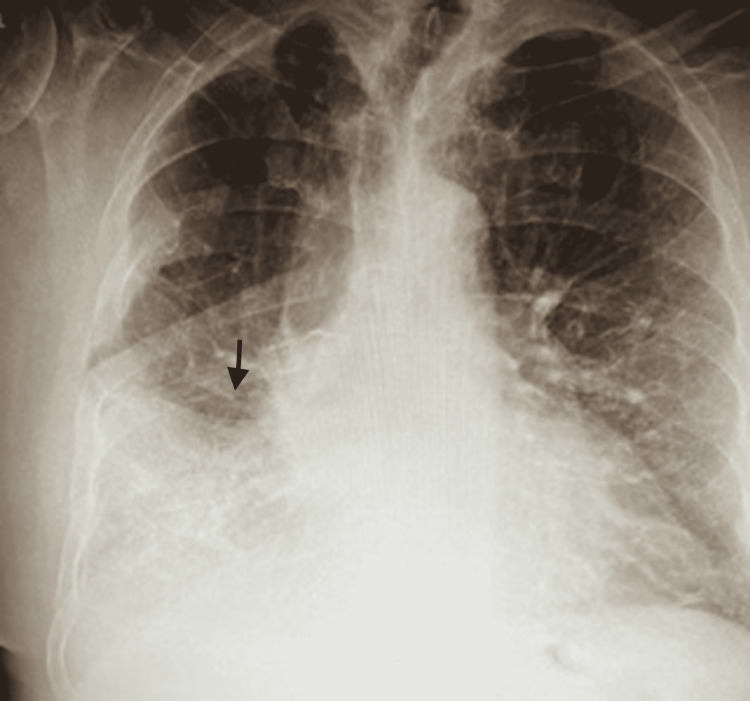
Chest x-ray demonstrating right lung opacity Arrow indicates the infiltrate
